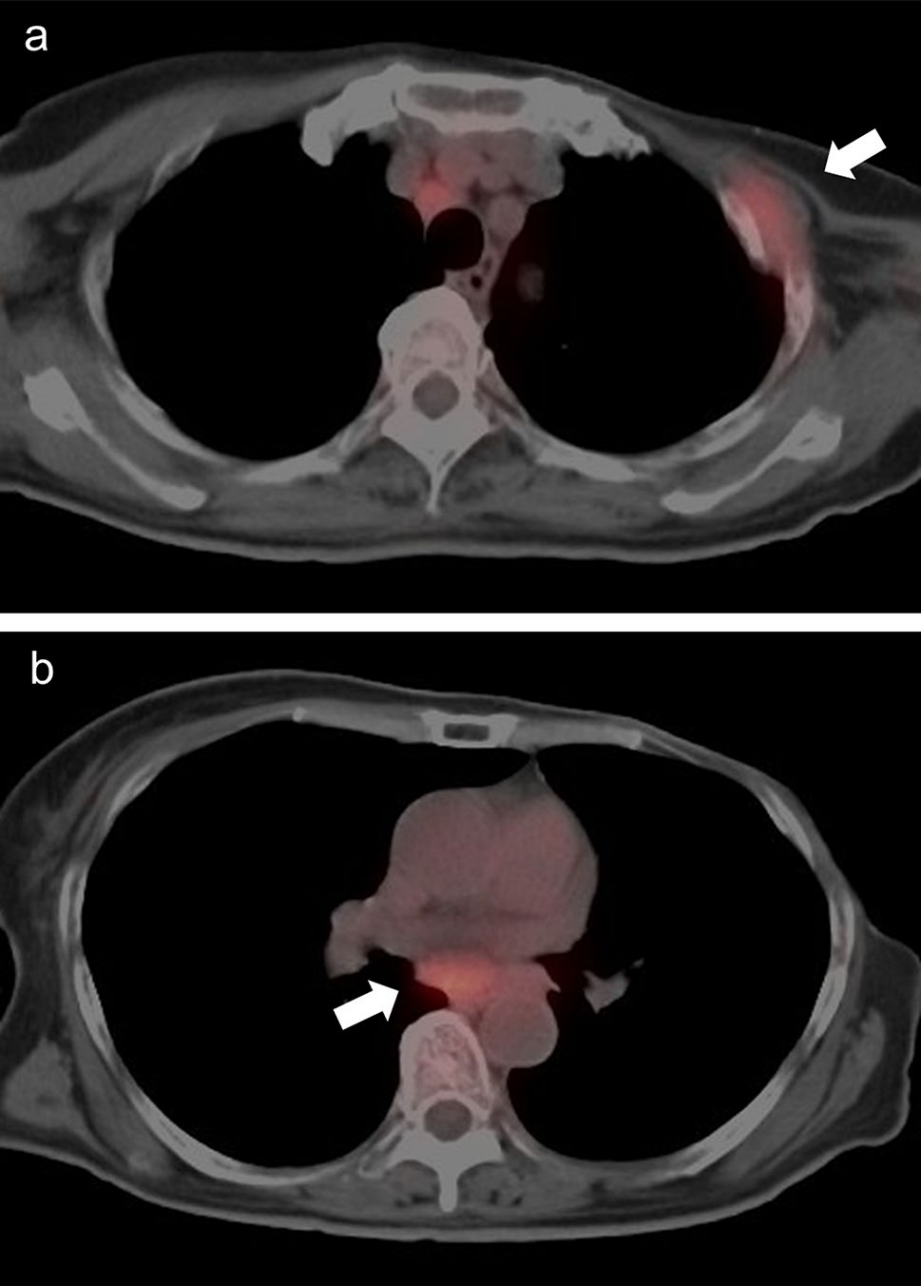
PET-CT shows abnormal uptakes in the left thoracic wall (a) and mediastinum (b) that indicate local recurrence at the thoracic wall and lymph node metastasis (see arrows)
